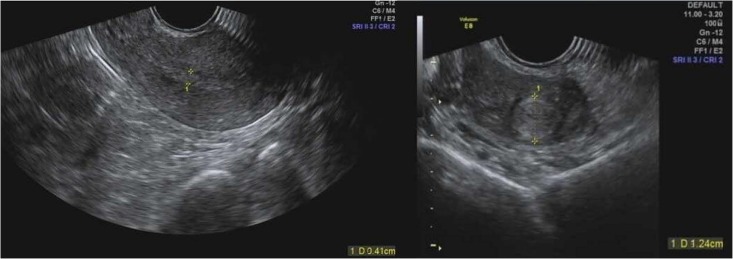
Uterus ultrasound pictures.
